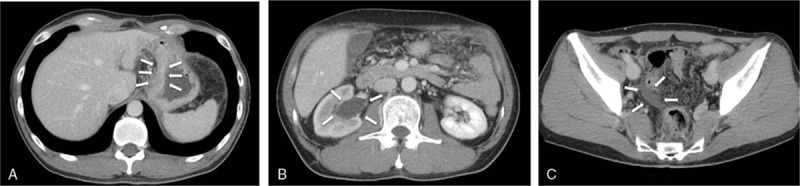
(A) Abdominal contrast-enhanced computed tomography (CT) suggested thickening of part of the posterior wall of the gastric corpus. (B) and (C). Right hydronephrosis and a small amount of ascites fluid were detected in the pelvic cavity.
